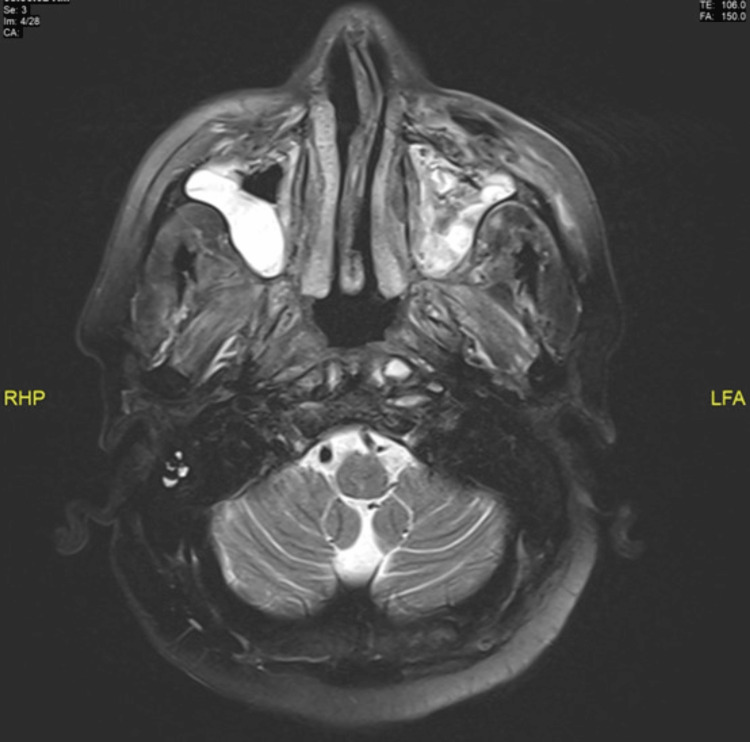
The magnetic resonance imaging (MRI) of the orbits and paranasal sinuses (PNS) showing marked mucosal thickening of the maxillary sinus.
